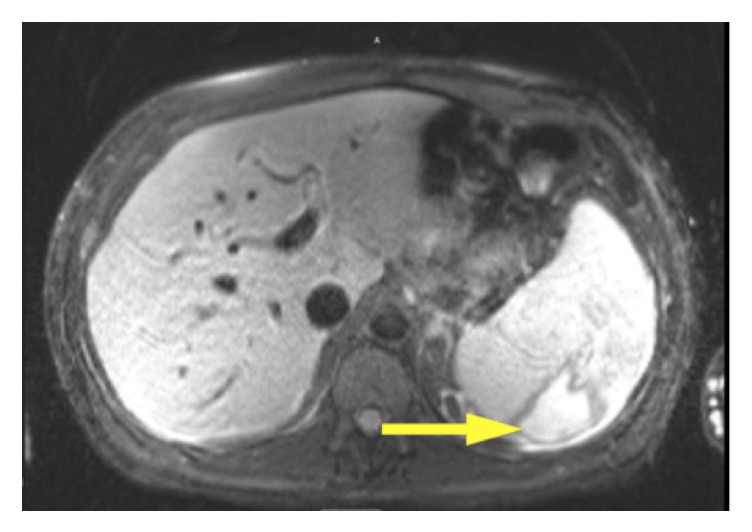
CT scan showing large splenic infarct.
